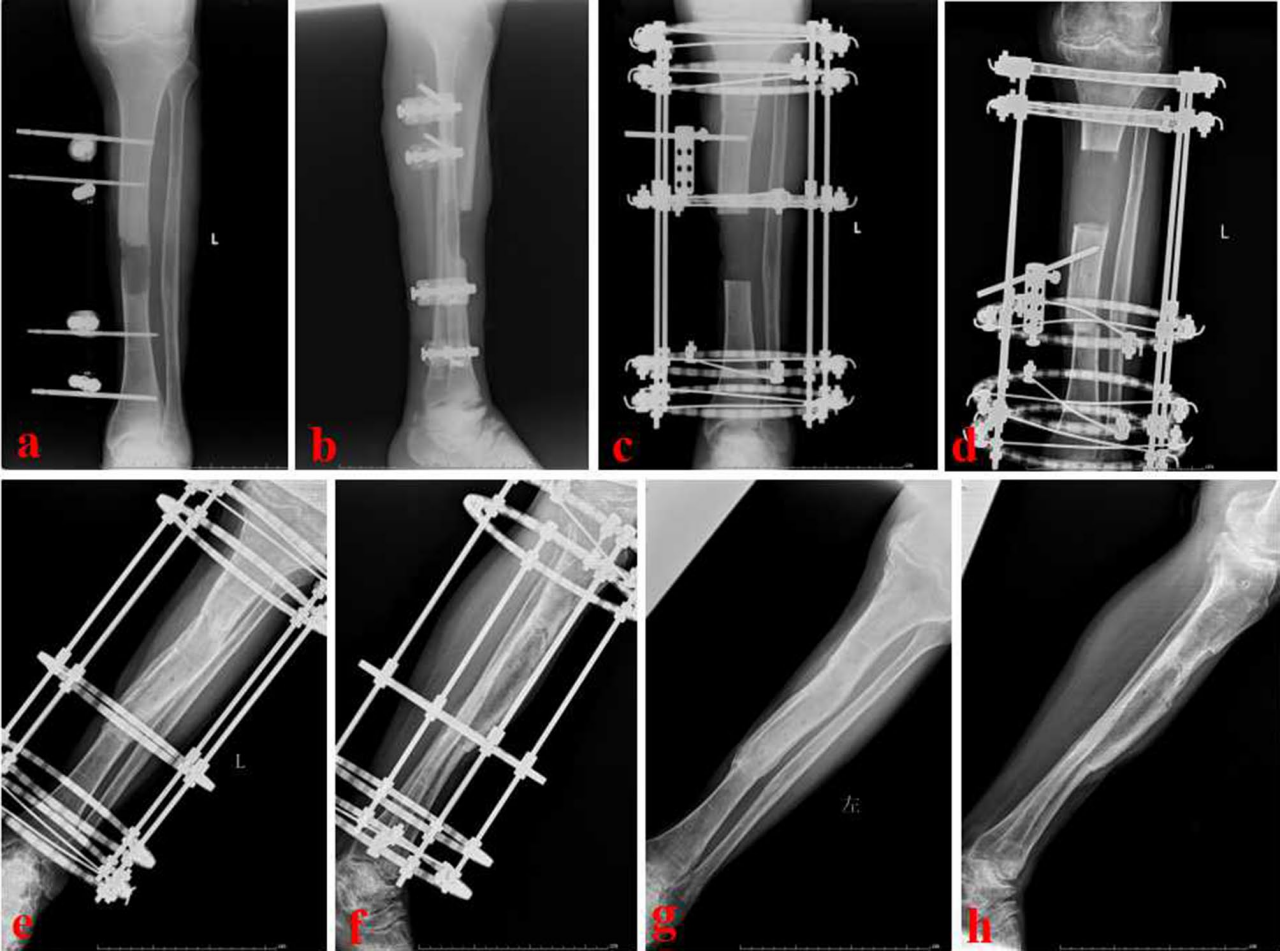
A 59-year-old male was successfully treated by the PBT technique. (a) and (b) The patient suffered from a severe infected tibial defects; (c) and (f) After radical debridement, the PBT technique was performed; (g) and (h): X-ray images showed that the bone defect was fully repaired. PBT: proximal bone transport.
